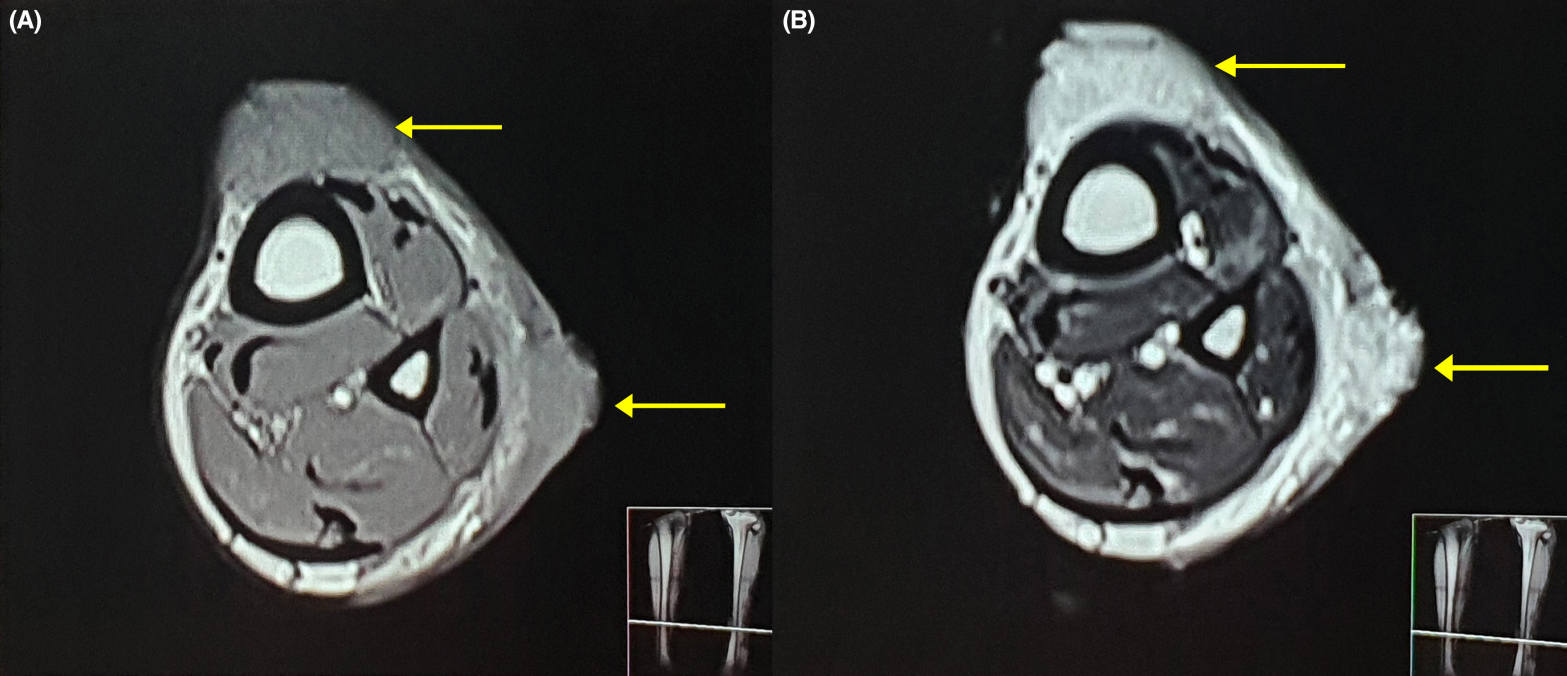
Magnetic resonance imaging of the lesion showing. (A) Iso signal intensity areas (arrows) in the subcutaneous plane on T1 weighted image of left lower leg on axial section. (B) Corresponding high signal intensity areas (arrows) in the subcutaneous plane on T2 weighted image of left lower leg on axial section.
